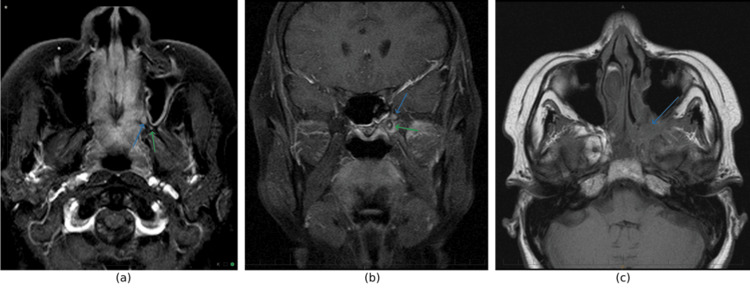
MRI demonstrating perineural spread of mucormycosis. (a) Axial post-gadolinium T1-weighted MRI demonstrating enlargement and enhancement of the greater palatine nerve (blue arrow) and lesser palatine nerve (green arrow). (b) Coronal T1-weighted post-contrast fat-saturated MRI demonstrating enlargement and enhancement of V2 within the foramen rotundum (blue arrow) and of the vidian nerve (green arrow), with abnormal enhancing tissue replacing the normal fat in the left pterygopalatine fossa, consistent with perineural spread. (c) Axial T1-weighted MRI demonstrating preserved normal fat in the right pterygopalatine fossa and pterygomaxillary fissure, in contrast to expansile intermediate-signal tissue replacing the fat within the left pterygopalatine fossa, in keeping with infiltrative fungal disease (arrow) MRI: magnetic resonance imaging
